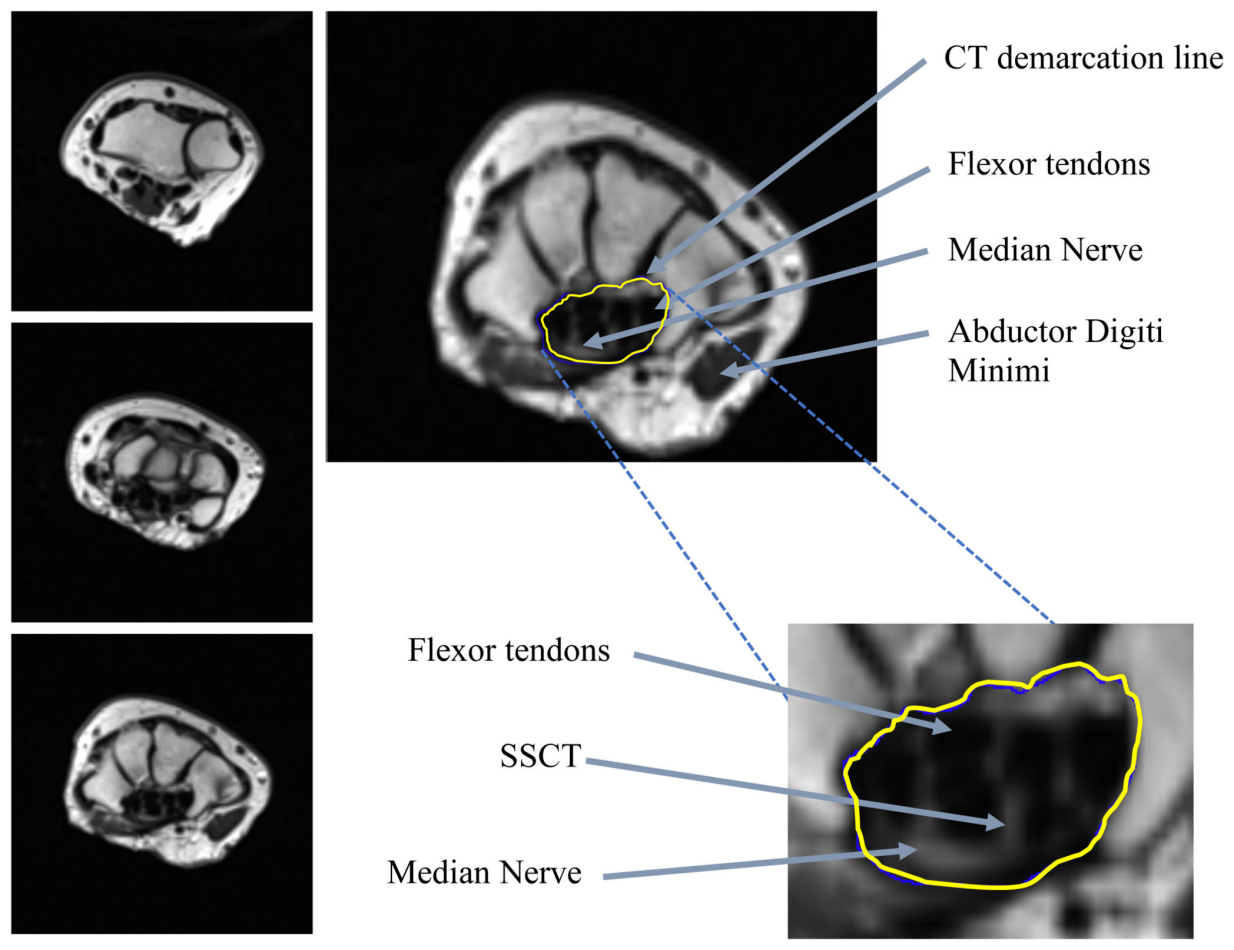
Left panel:* T2-weighted MRI at the proximal wrist landmark (top image), middle landmark (middle image), distal landmark (bottom image). *Right panel:* Top: T2-weighted MRI with the carpal tunnel demarcated at distal landmark (yellow line). Labels demonstrating median nerve, flexor tendons, and abductor digiti minimi (used for intensity normalization). *Bottom:* ‘zoomed-in’ image showing SSCT visible as tissue surrounding flexor tendons and median nerve within the demarcated carpal tunnel region.
